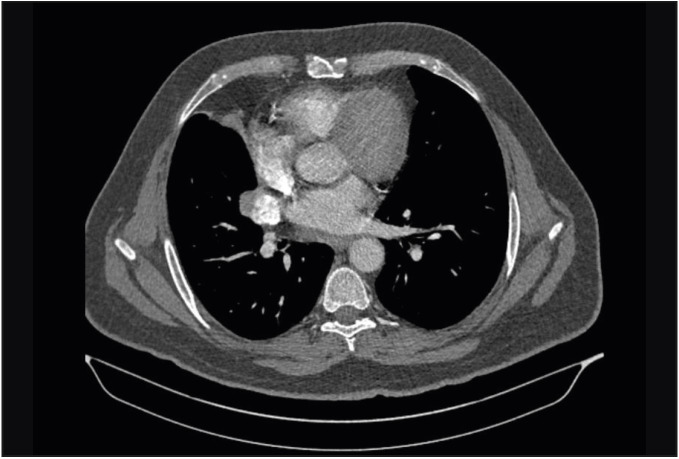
Contrast-enhanced thoracic CT scan; mediastinal window. showing a mass of 37x30 mm in diameter, which results in a complete obstruction in the right middle lobe bronchus, leading to an obstructive atelectasis of the middle lobe.
